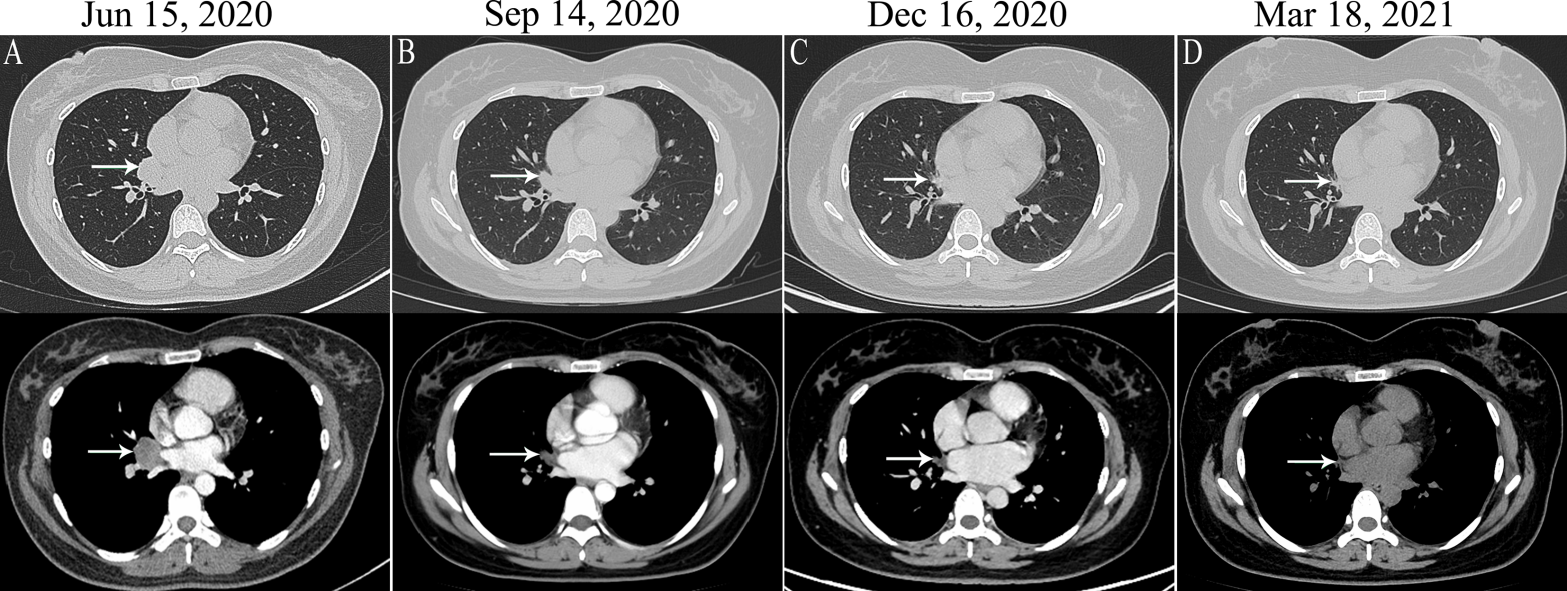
The lung target lesion on CT (white arrow). (A) Computed tomography (CT) scan showed a 2.7 cm×2.5 cm mass in the hilum of the right lung before treatment. (B) the imaging of lung target lesion after 1 month of alectinib treatment (1.4 cm × 1.1 cm lesions). (C) the imaging of lung target lesion after 4 months of alectinib treatment (1.2 cm × 1.0 cm lesions); (D) the imaging of lung target lesion after 7 months of alectinib treatment (1.1 cm × 0.7 cm lesions).
